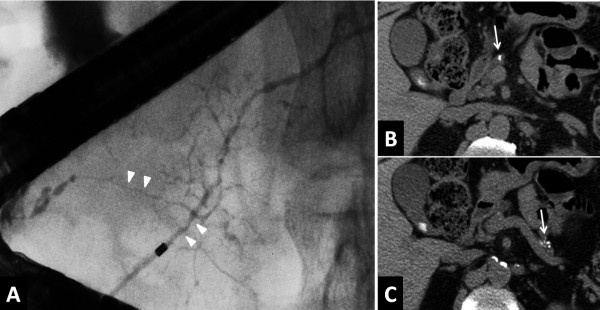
ERCP and CT findings in a 69-year-old man with narrowing of both Wirsung’s and Santorini’s ducts. (A) ERCP at diagnosis in April 2001 showing Wirsung and Santorini duct narrowing (arrowheads). (B), (C) CT 105 months later in December 2009 showing pancreatic stone formation and pancreatic atrophy (arrow). Data are reprinted from Ref. [41] with permission from the Journal of Gastroenterology.
